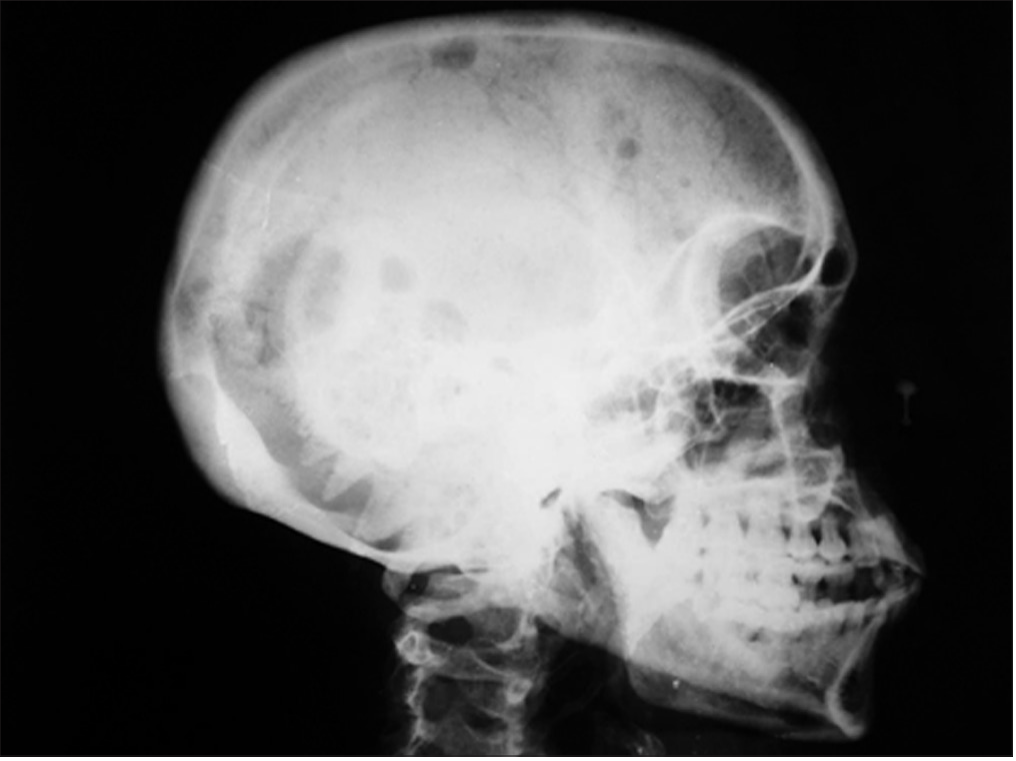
Skull radiograph revealing multiple lytic lesions
